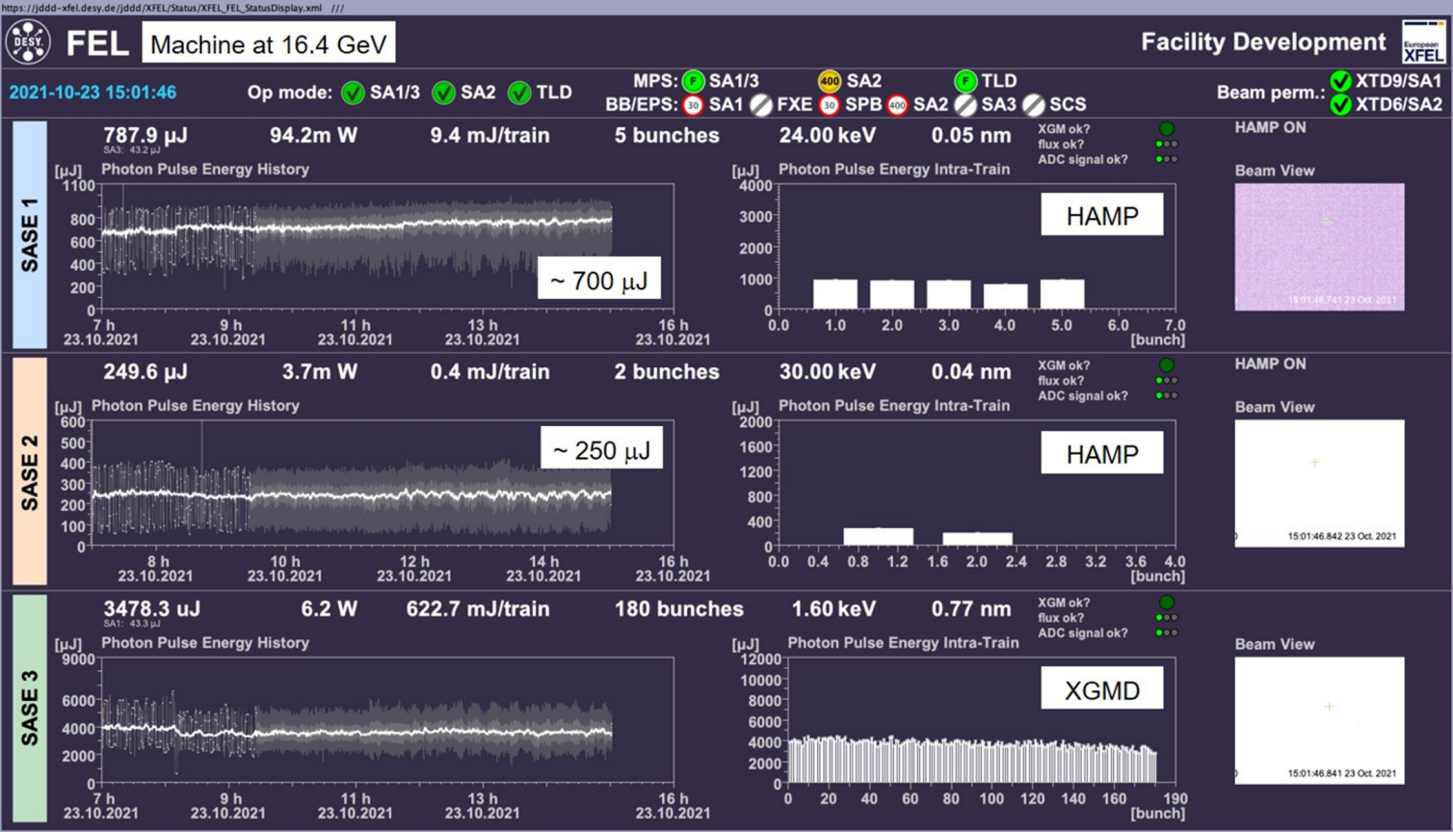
EuXFEL operation at very hard X-rays: single-shot upstream XGM detectors for SASE1 and SASE2 were HAMP (Xe gas target), whereas SASE3 was operated with XGMD (Kr gas target). The electron bunches were accelerated to 16.4 GeV. The SASE1, SASE2 and SASE3 photon energies were 24 keV, 30 keV and 1.6 keV, respectively. Left: graphs are the arithmetical averages over the single-shot pulse energies. Right: actual single-shot pulse energies plotted as bars. The average light powers at all SASE sources are shown.
